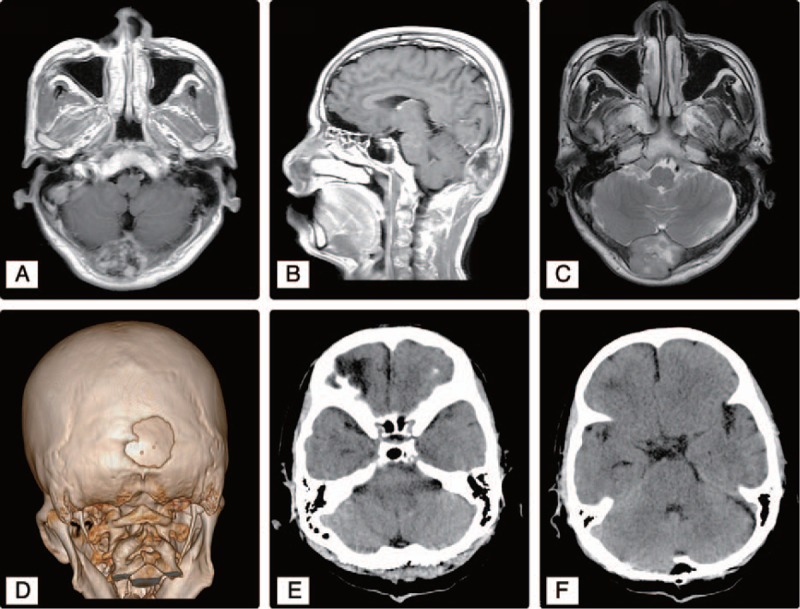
Imaging findings. A–C, Preoperative brain T1 magnetic resonance imaging (sagittal and axial images, respectively) reveals a heterogeneous enhanced mass, measuring approximately 3 cm × 3 cm × 2 cm. The tumor has clear boundaries with the bone tissue, invades the dura mater, compresses the brain tissue, and shows osteolytic changes in the bone. D, Preoperative three-dimensional computed tomography of the skull shows a defect in the bone window of approximately 2.5 cm × 2.5 cm, with invasion to the whole skull layer. E, F, After resection of the mass and surrounding skull, a titanium plate has been used to repair the defect in the skull.
